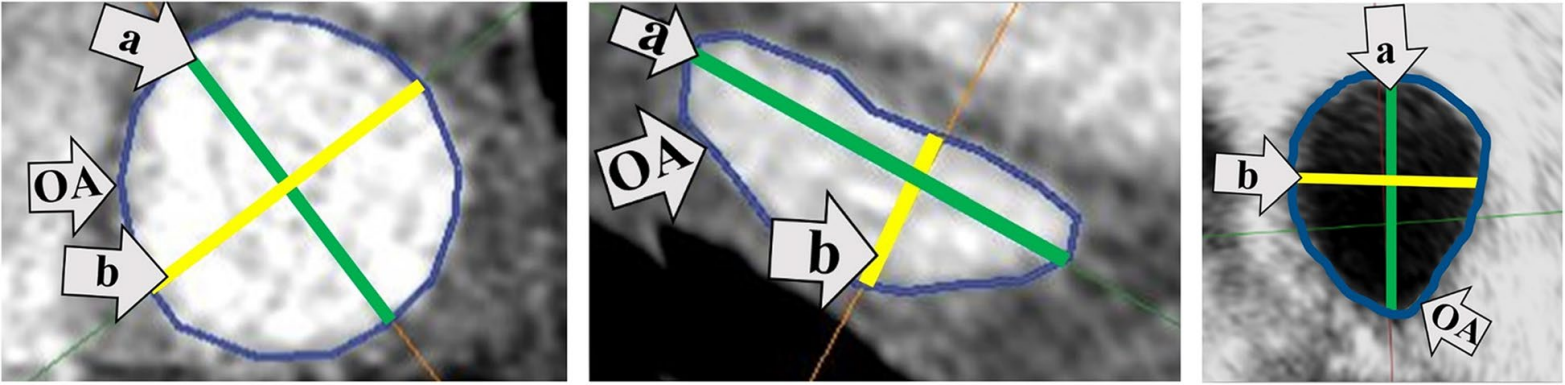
Measurement of PV ostium parameters. 2a left: Measurement of major (a, green line) and minor axis (b, yellow line) PV diameters as well as planimetric measurement of OA (blue line) from the „en face” 2D plane of a CCT image in case of a circular PV ostium. 2b middle: Measurement of the same parameters on a CCT image in case of an elliptic ostium. 2c right: Measurement of the same parameters on a 3DTOE image. PV (Pulmonary vein), a (The major axis diameter of the PV ostium), b (The minor axis diameter of the PV ostium perpendicular to „a”), *OA* (Ostium area), *2D* (Two-dimensional), *CCT* (Cardiac computed tomography), *3DTOE* (Three-dimensional transesophageal echocardiography)Width of the LLR and the carina (Fig. 3):
